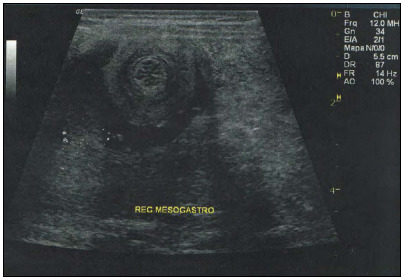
Abdominal ultrasound image showing an onionskin-like part of the small bowel suggestive of intussusception.
